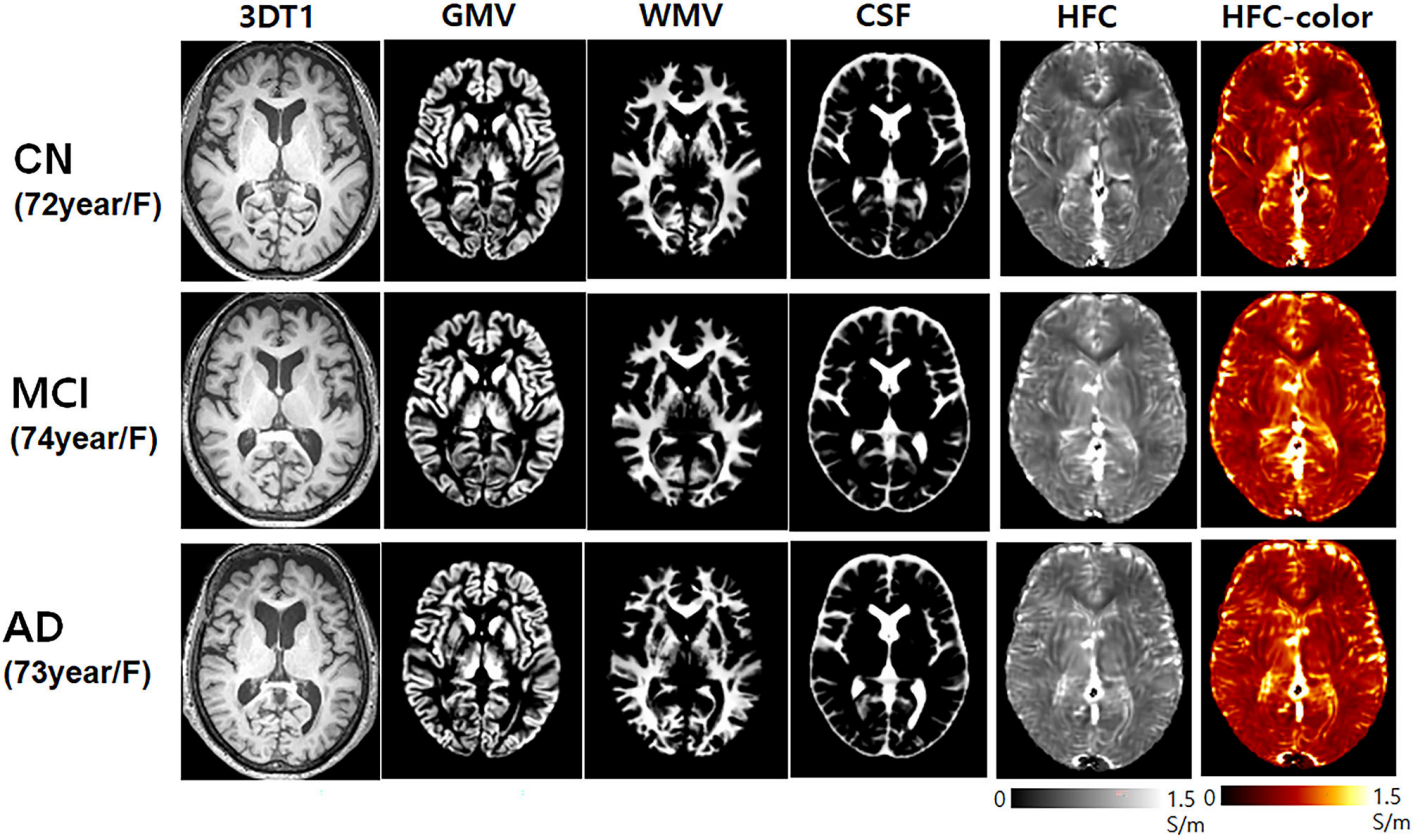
Representative high-frequency conductivity (HFC) maps and the 3D T1-weighted image with the corresponding segmented brain tissue volumes of gray matter and white matter obtained from one cognitively normal (CN) elderly (72-year-old female), one mild cognitive impairment (MCI) (74-year-old female), and one Alzheimer's disease (AD) (73-year-old female) participants.
